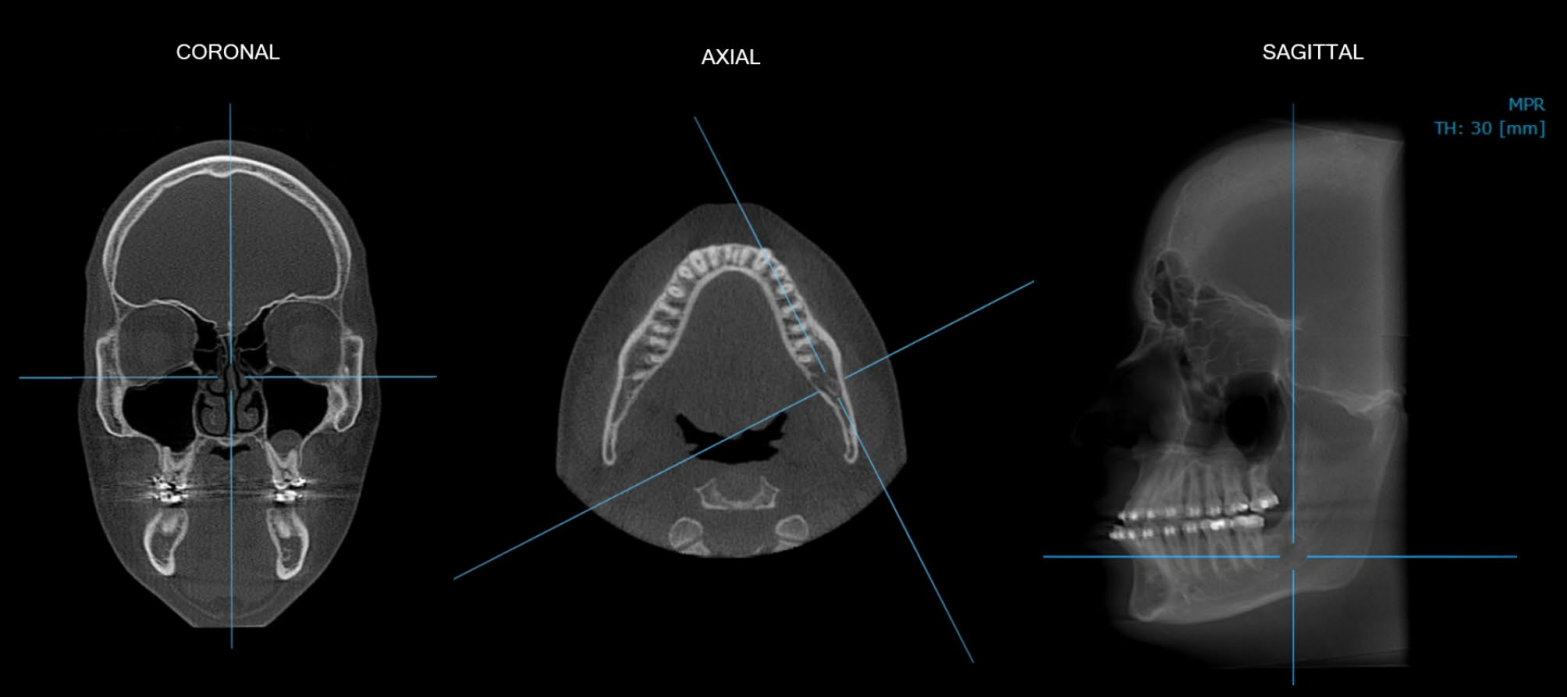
Spatial reorientation of the CBCT multiplanar reconstructions to standardize the assessments of linear measures of the mandible angle
